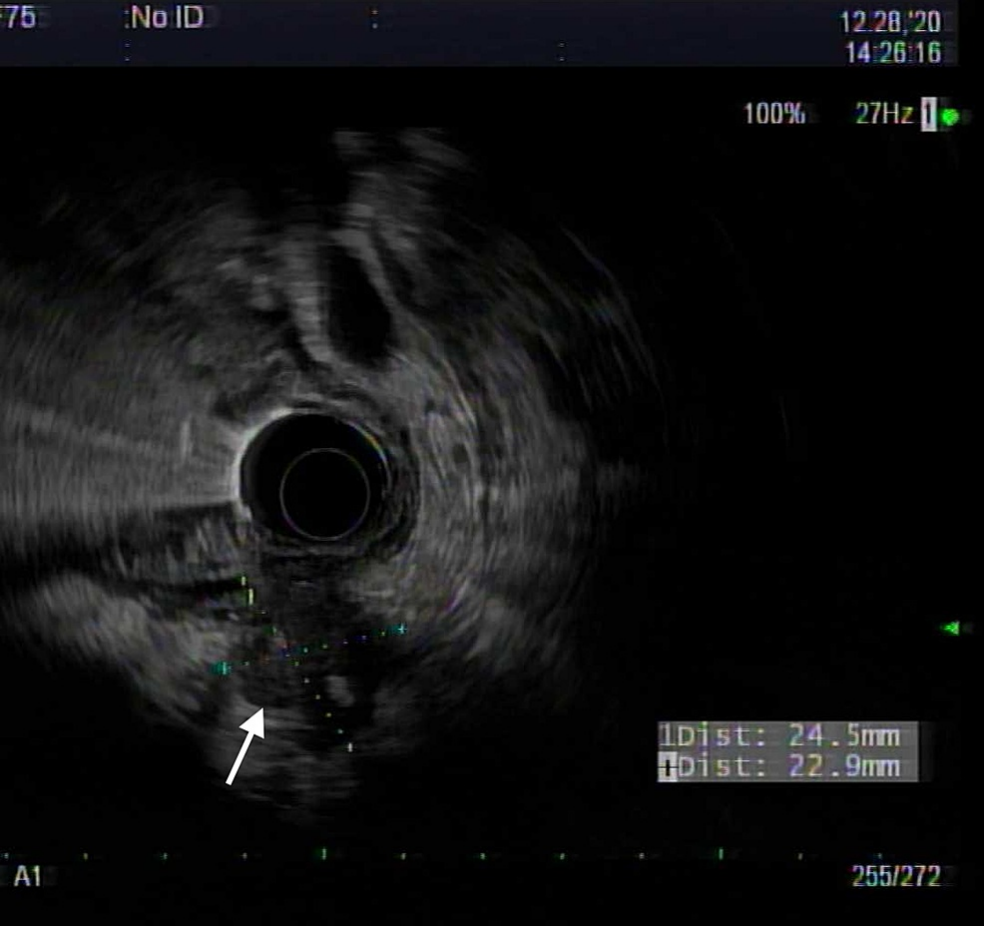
Esophageal carcinoma seen on endoscopic ultrasound (EUS) evaluation
